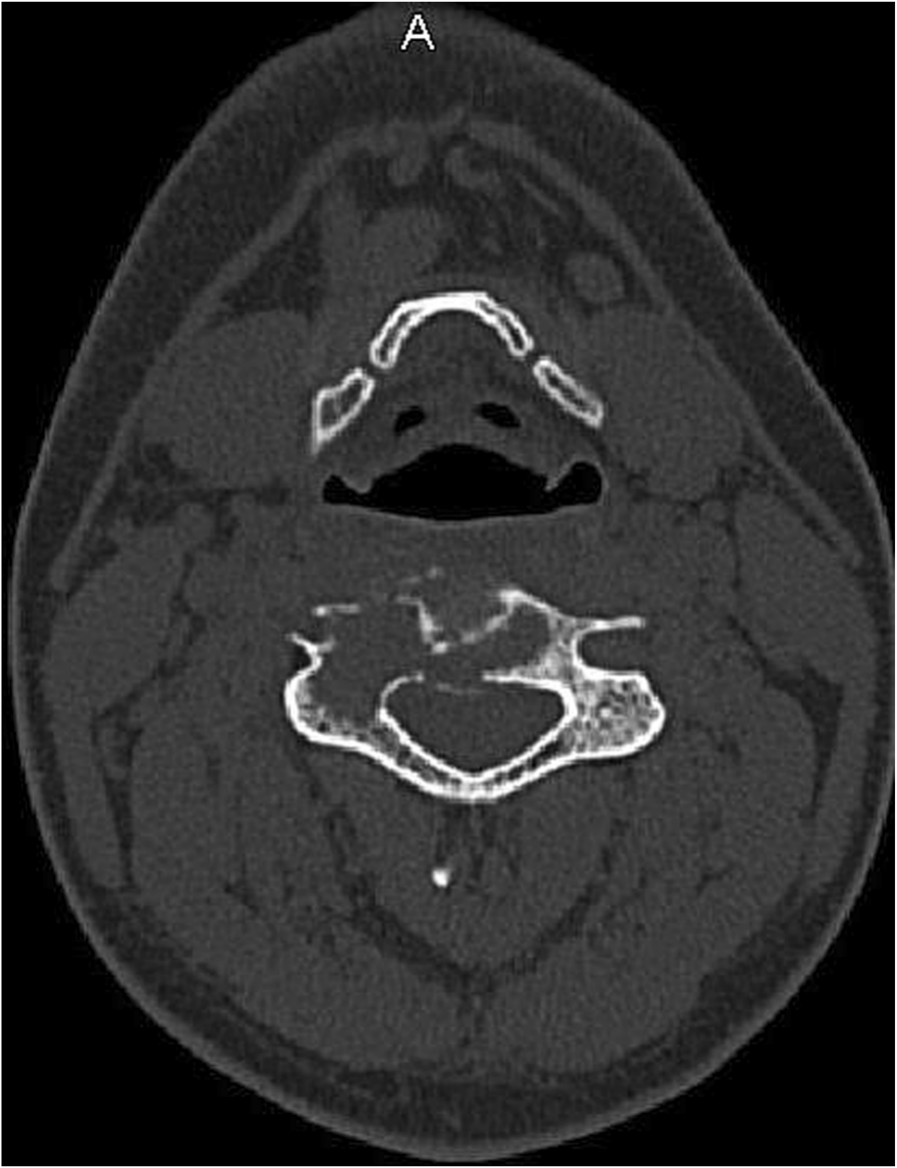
Lesion extending from the vertebra corpus to the lateral mass on CT in the axial plane
